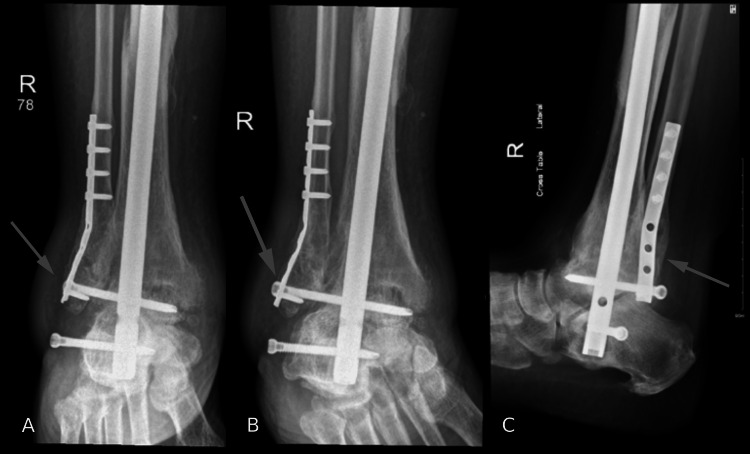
(A) Anteroposterior, (B) oblique, and (C) lateral X-rays of the right ankle joint on the latest follow-up
